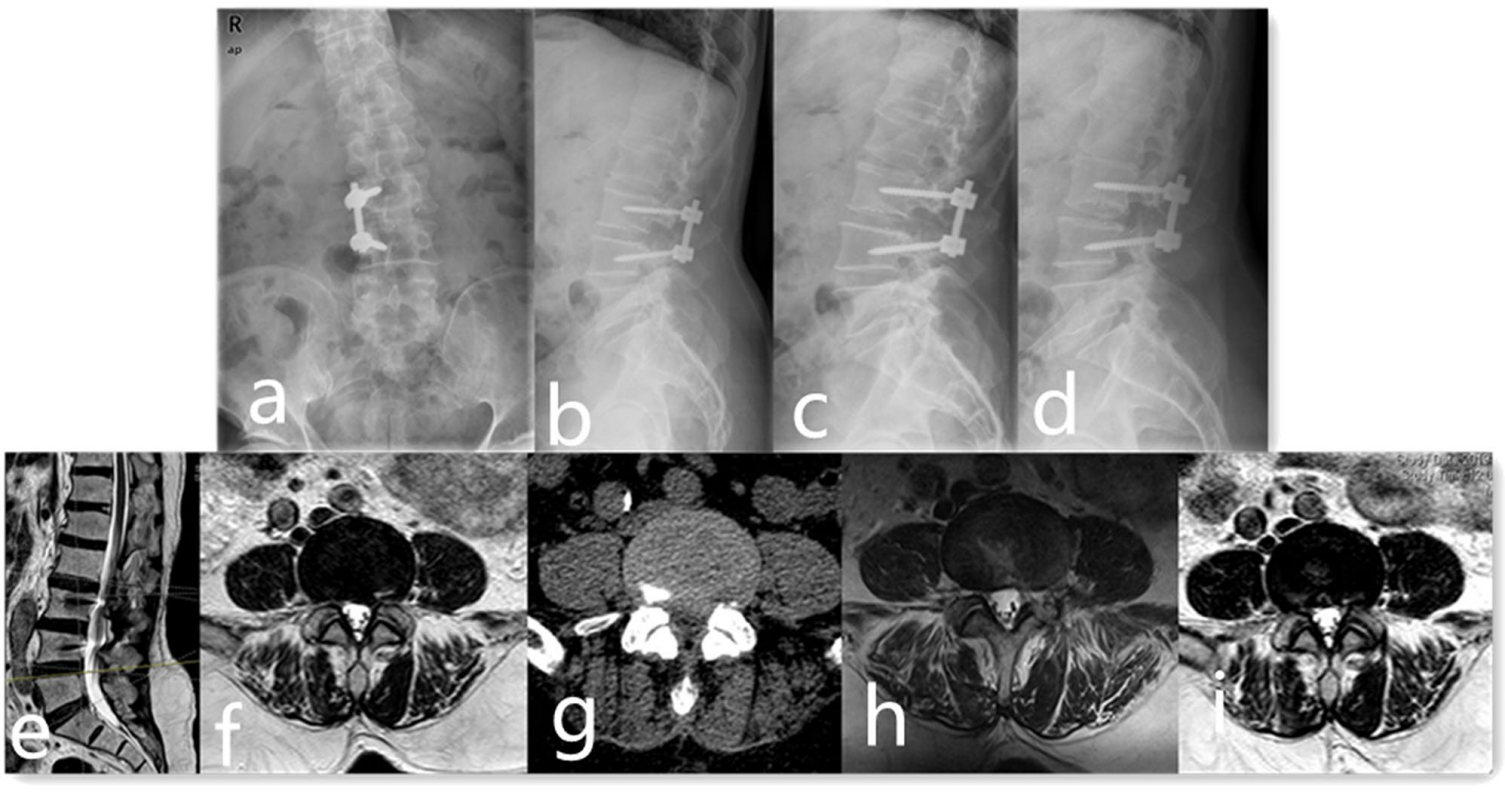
A 66-year-old female was diagnosed as symptomatic ASD at the L4/5 level. He was performed with posterior decompression and internal fixation at L3-4 due to disc herniation 3 years ago. a, b, c, d Preoperative anterior-posterior, lateral and dynamic lumbar X-ray showed posterior fixation with an intervertebral cage at L3/4 and no instability was found at L4/5. e, f, g Preoperative MRI and CT showed disc herniation at L4/5, which compressed L5 left nerve root. h Postoperative MRI showed percutaneous transforaminal endoscopic lumbar surgery was performed at the left side of L4/5 and protruded disc was removed. i Postoperative MRI at 1-year follow-up showed good results were achieved and no disc herniation was found
